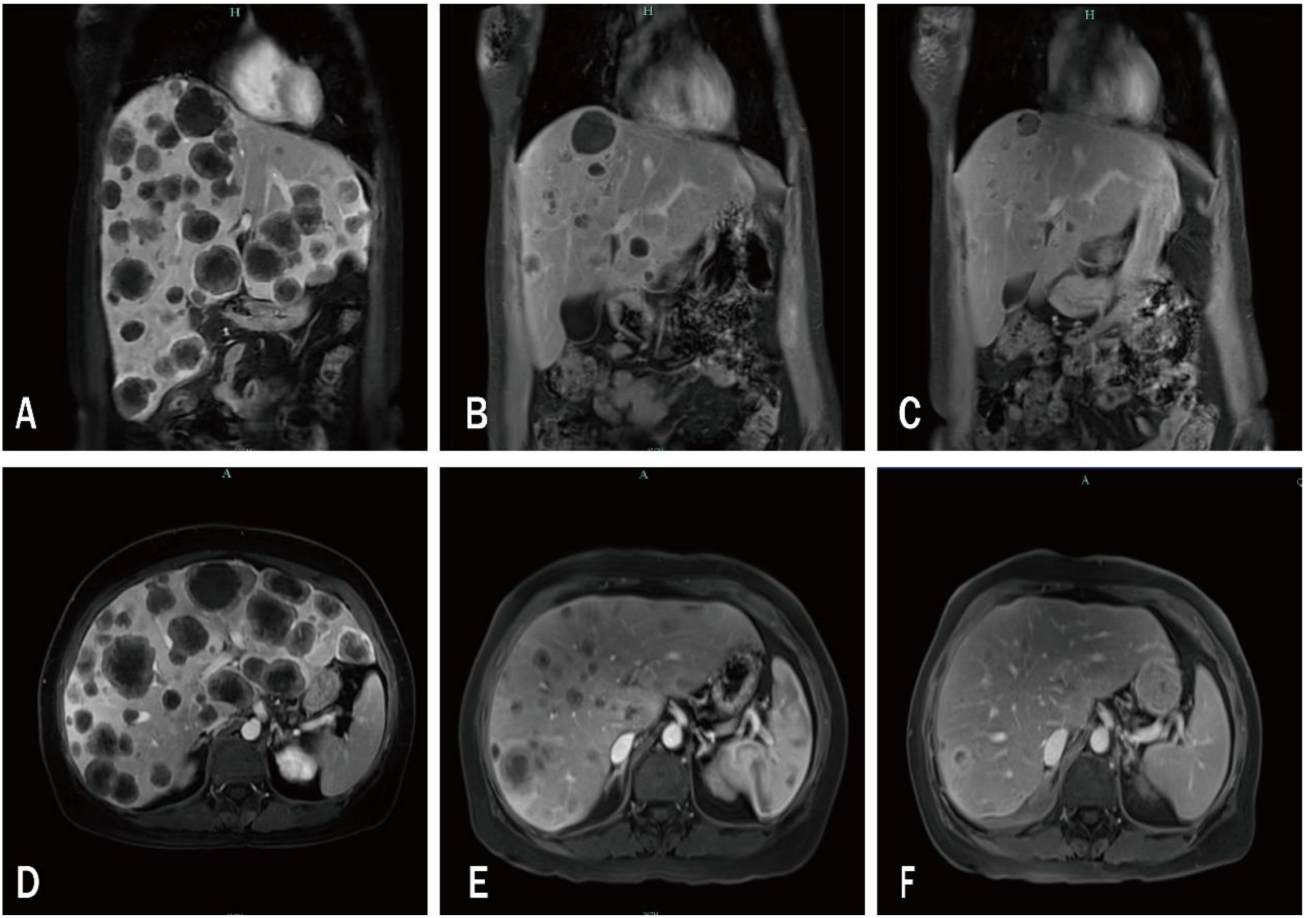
Serial T1-weighted magnetic resonance images of the upper abdomen. (A, D) baseline scan (9 April 2024) showing multiple intra-hepatic metastases; (B, E) follow-up after two treatment cycles (26 May 2024) demonstrating marked tumor regression; (C, F) subsequent follow-up (31 July 2024) revealing continued reduction in liver metastatic burden.
